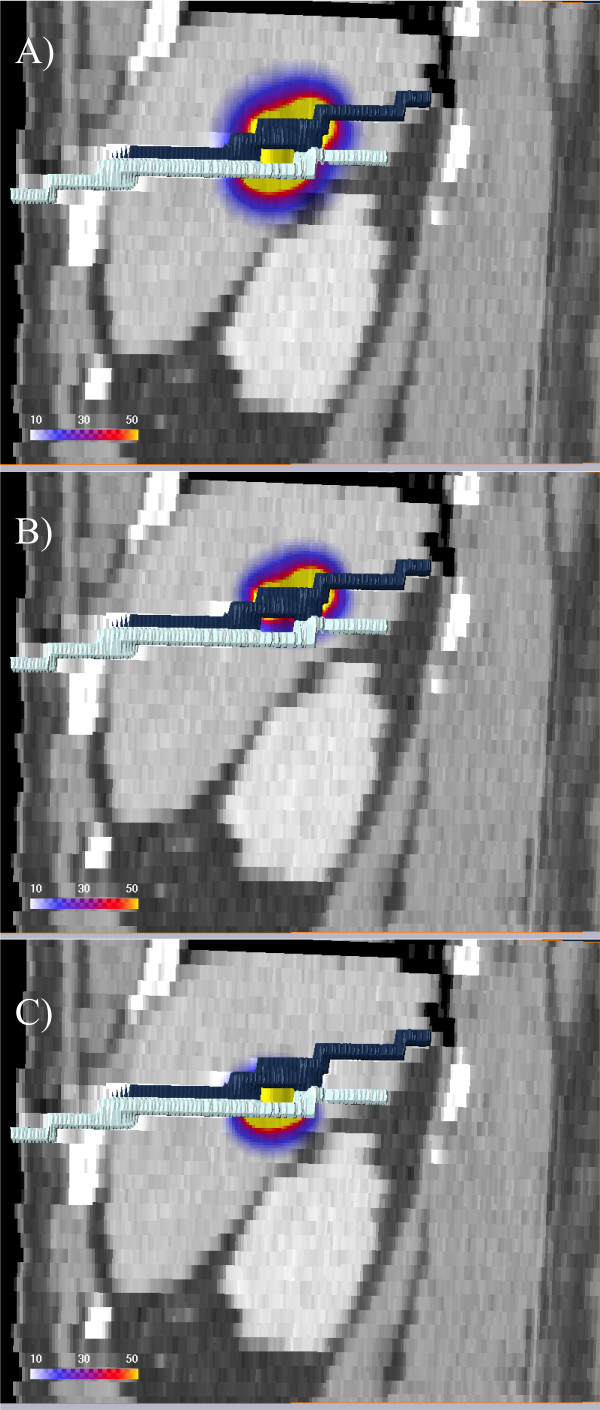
Dose separation. The 3D visualization shows a coronal CT reconstruction with the calculated dose in Gy overlayed using the patient in Fig. 1. The dose is applied using two catheters. The two catheters were visualized in 3D using surface rendering of the catheters labeled in the CT scan. A) Total dose, *D_tot _*, overlayed. B) Dose applied by the cranial catheter, *D*_1_. C) Dose applied by the caudal catheter, *D*_2 _.
